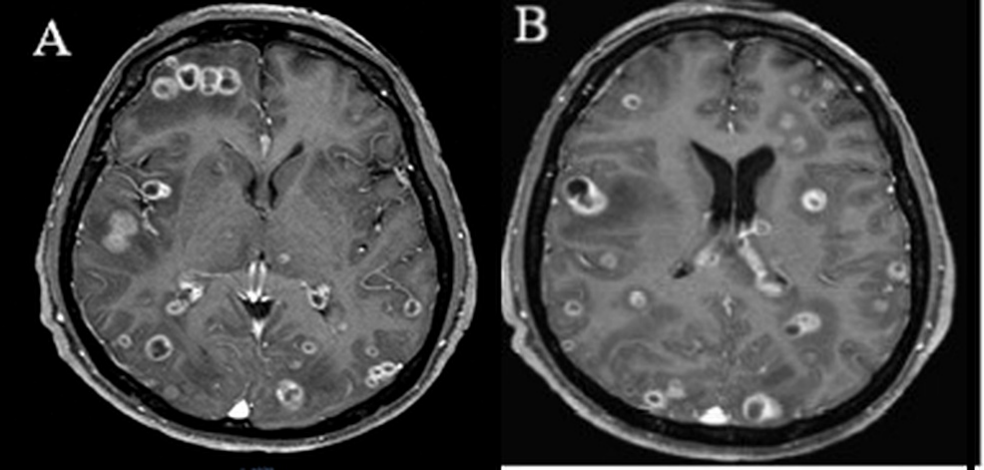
Axial T1-weighted magnetic resonance imaging (MRI) with contrast. (A) Multiple parenchymal lesions with ring enhancement in the right frontal, occipital lobes, and mesencephalon, with vasogenic edema; and (B) superior transversal image showing multiple ring-enhancing lesions, the parietal and occipital lobes are the most affected.
